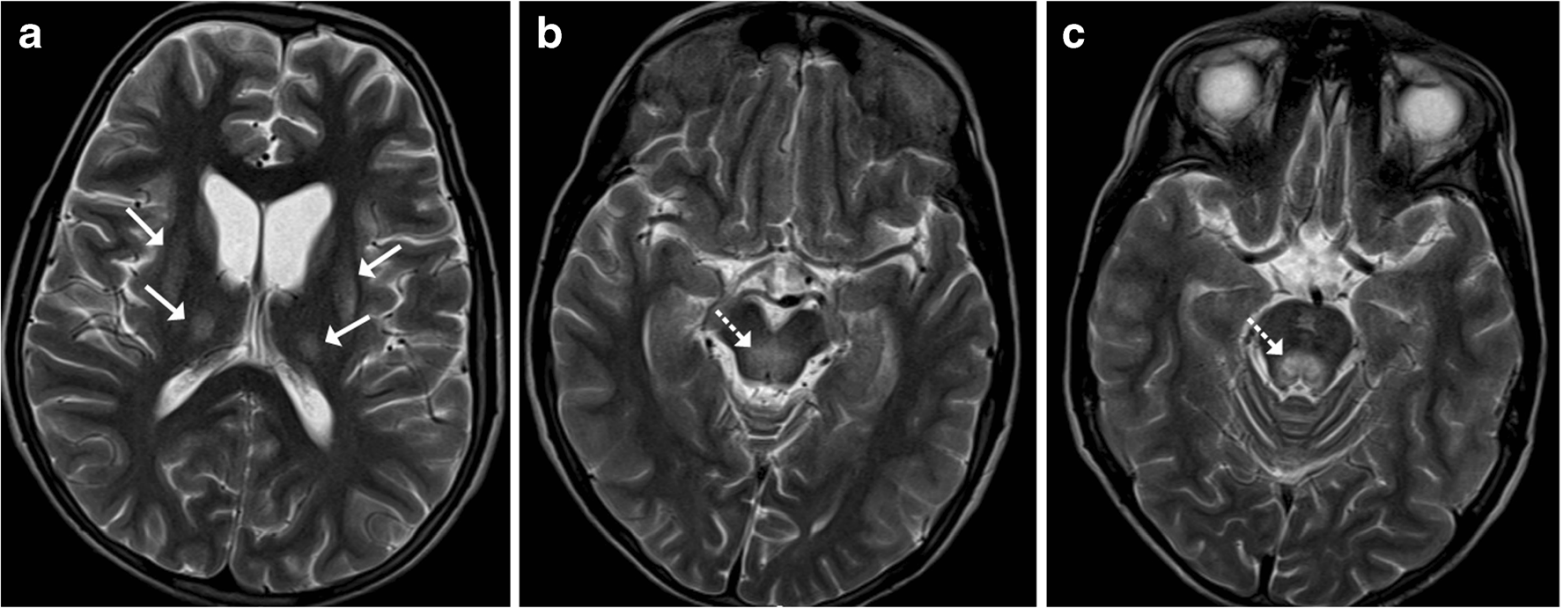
A 9-year-old patient known to have Wilson’s disease: a Axial T2-weighted image shows symmetrical atrophy of the basal ganglia with hyperintense signal involving the basal ganglia and thalamus (*arrows*). b–c Axial T2-weighted image shows increased signal intensity involving the midbrain as well as the pontine tegmentum (*dotted arrows*)
